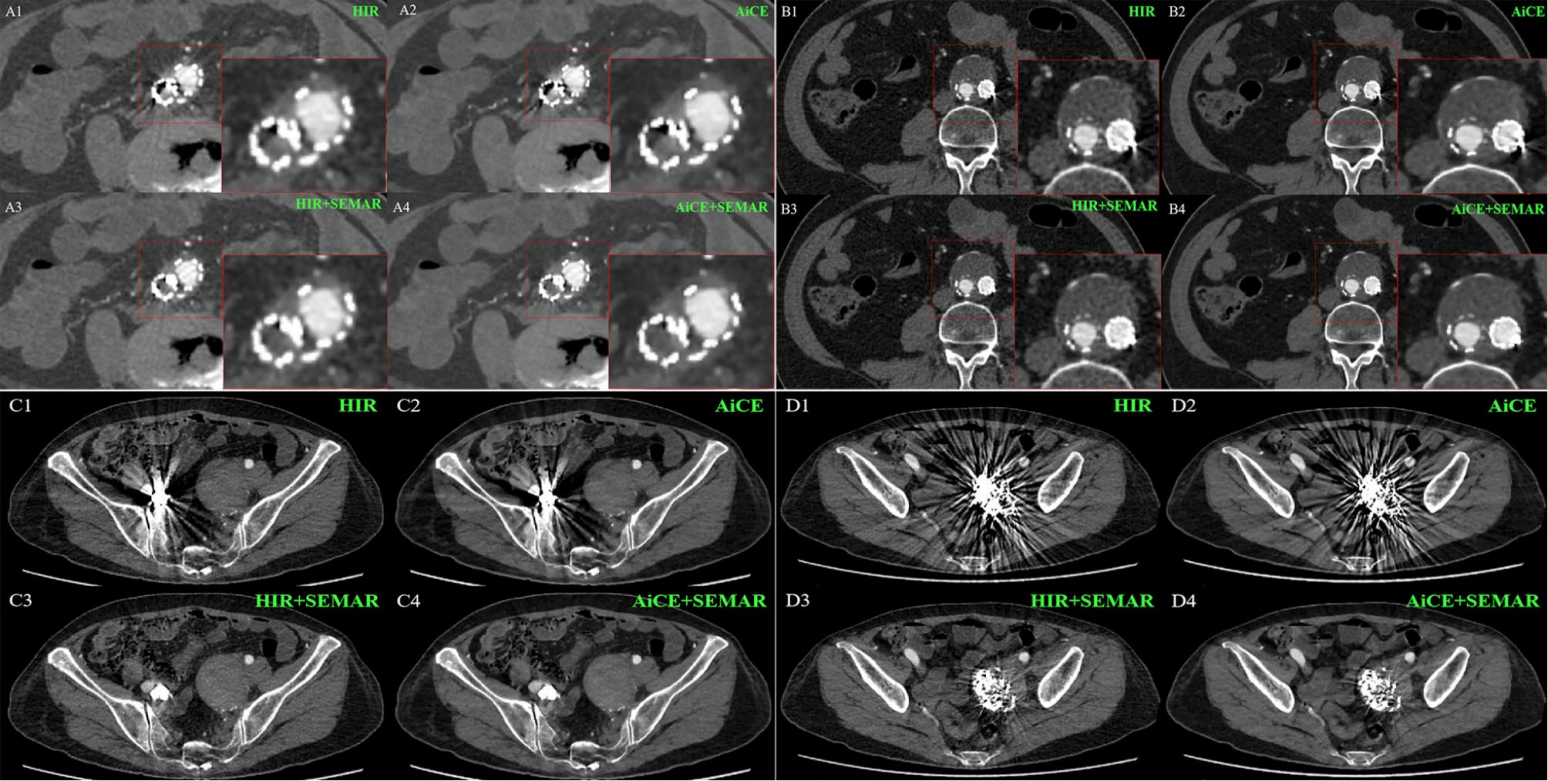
Exemplary axial CTA images with different reconstructions for qualitative evaluation. (A1-A4) A 73-year-old woman. Reader 1 and 2 scored the degree of in-stent vessels on the most severe artifact scan as 1/1/3/3. (B1-B4) A 66-year-old man. The degree of in-stent vessels on the most severe artifact scan was rated as 1/1/3/3 by readers 1 and 2, and the degree of out-stent thrombus on the most severe artifact scan was rated as 2/2/4/5 by readers 1 and 2. (C1-C4) A 70-year-old woman. Both Reader 1 and Reader 2 scored the degree of artifacts on the most severe coil artifact scan as 1/1/5/5. (D1-D4) A 70-year-old woman. The degree of artifacts on the most severe coil artifact scan was rated as 1/1/3/4 by Reader 1 or 1/1/4/4 by Reader 2. The block in the lower left corner of a single image (A1-A4, B1-B4) represents the local amplification of the respective places
